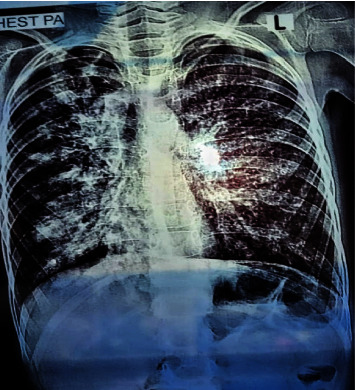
X-ray imaging of the patient reveals characteristic pulmonary manifestations, including hyperinflation, bronchiectasis, and mucus plugging, reflect the disease's impact on airway structure and function.
